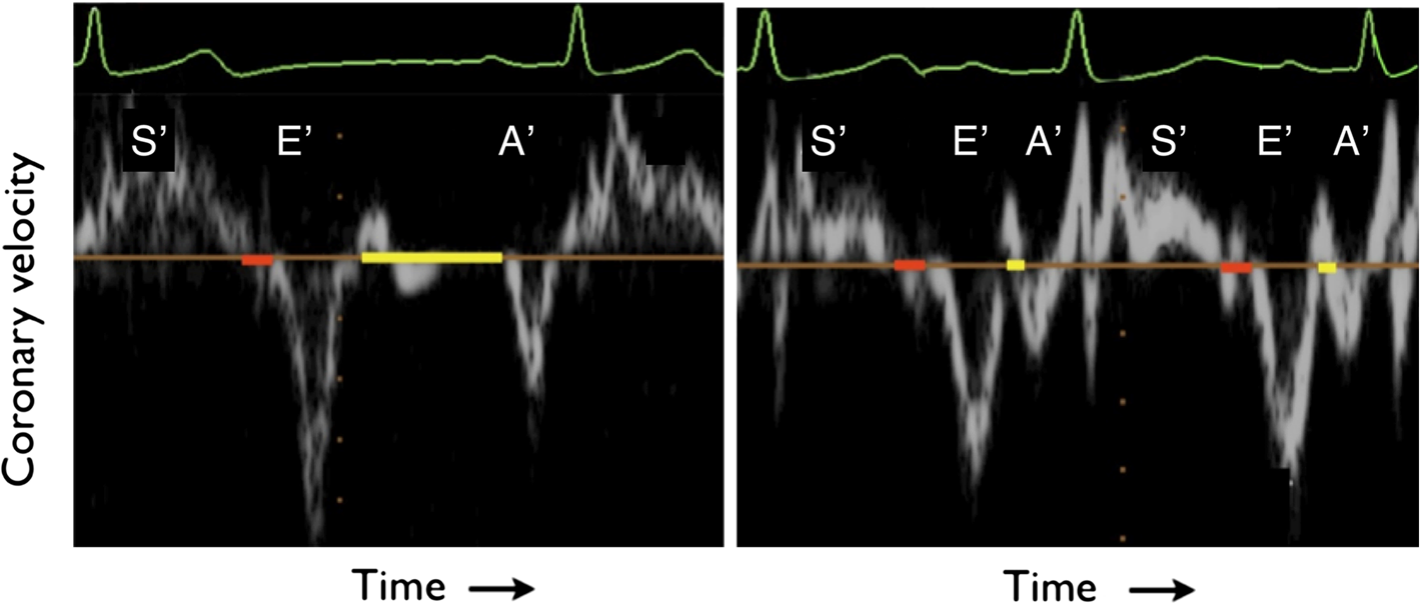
Tissue Doppler waveforms of the left atrio-ventricular groove (circumflex artery) at low (left) and high (right) heart rate. Coronary motion occurs throughout systole (S’). At the end of systole there is a short period of relative stasis between the closing of the aortic valve and the opening of the mitral valve (isovolumic relaxation time, IVRT), shown in red. This period varies little with heart rate. The period of minimal cardiac motion (yellow band) occurs between the E’ and A’ waves. The PMCM shortens with increasing heart rate. Images have been resized and edited for clarity.
